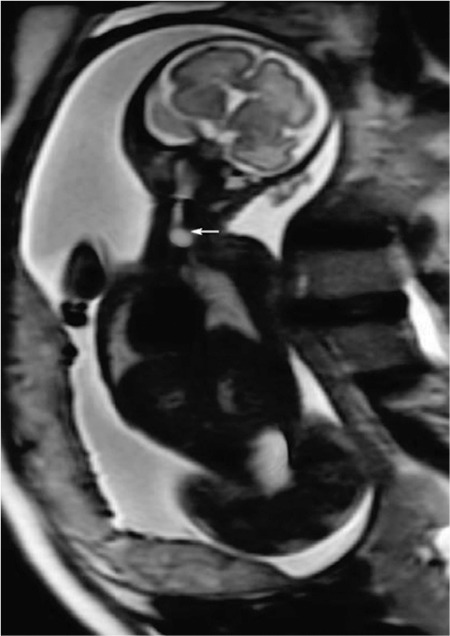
T2W1 magnetic resonance imaging shows the upper paraesophageal pouch.
